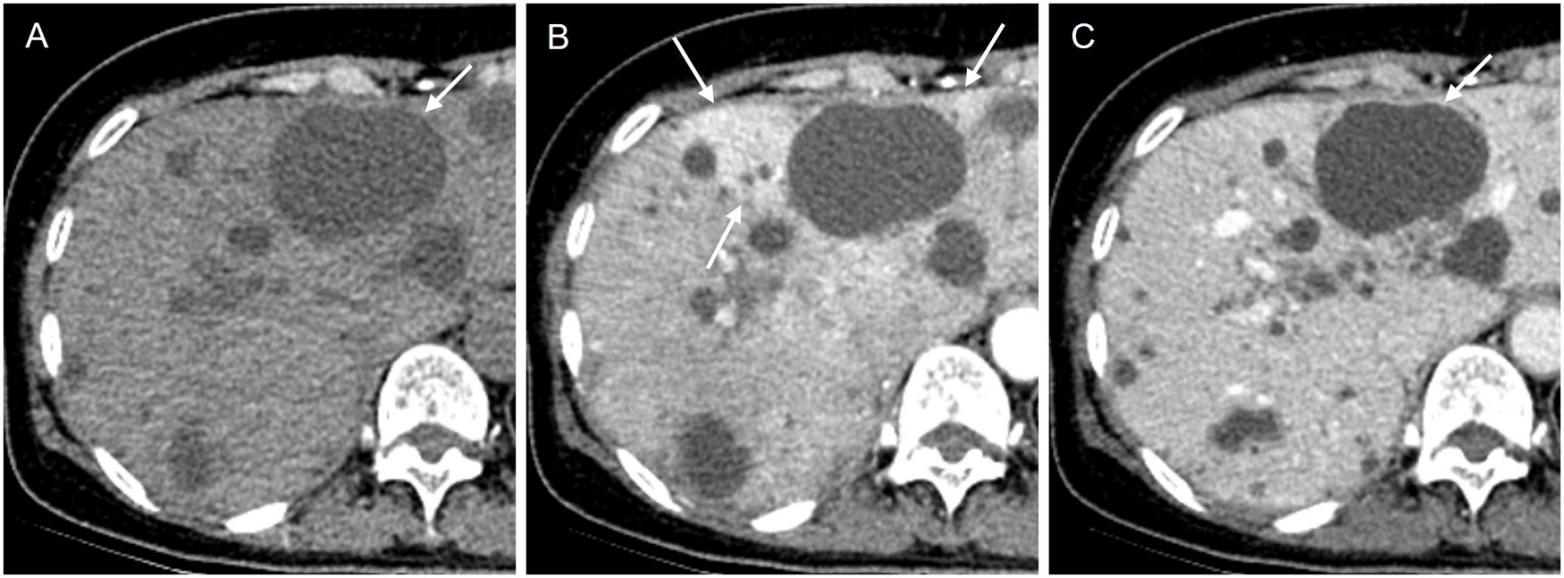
A 65-year-old female patient with an infected cyst (H10 in Fig 2). (A) On the pre-contrast CT image, a proven 6 cm infected cyst (arrow) shows no remarkable finding. (B) On the arterial phase image, there is perilesional hyperemia around the cyst (arrows). (B, C) On the arterial or delayed phase image, no discernible wall thickening is noted. In this case, arterial hyperemia is the only clue for cyst infection.
